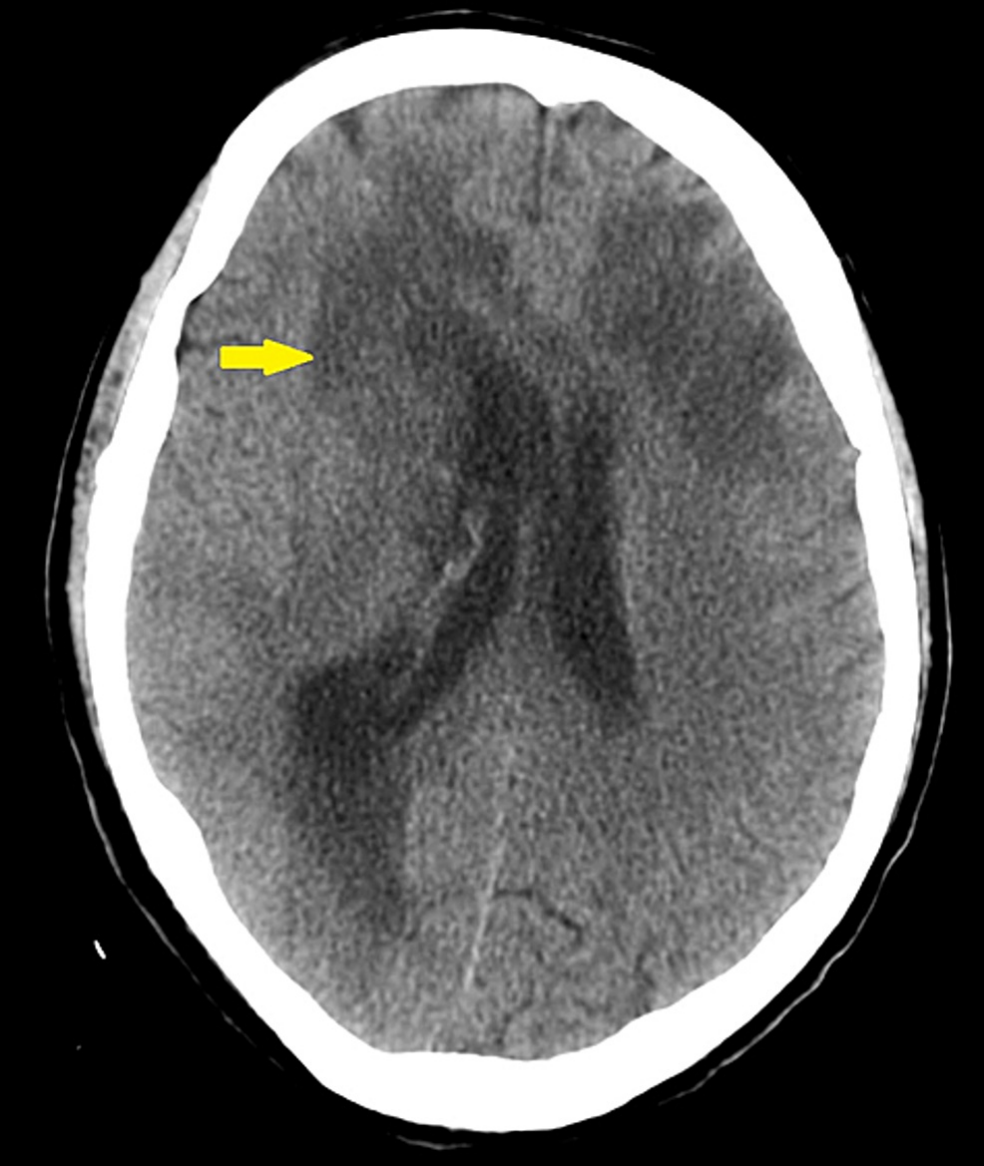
Computed tomography (CT) scan of the head without intravenous contrast with findings concerning the development of a brain abscess particularly on the right where there was mass effect, midline shift, and occlusion of the right lateral ventricle with mild dilatation of the right lateral ventricle.
